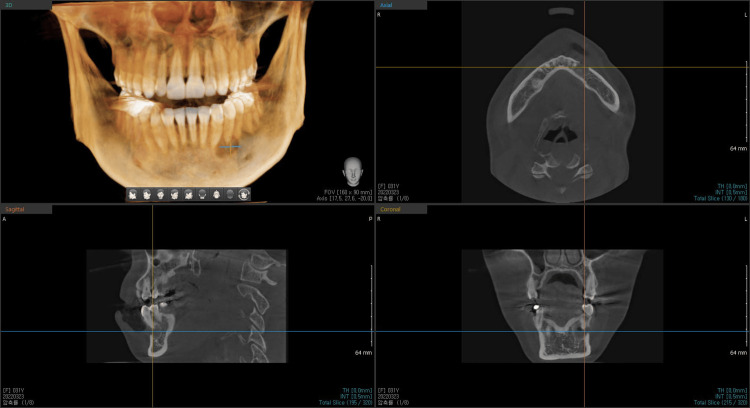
Dental cone beam computed tomography (CBCT) of the lesion 3D reconstruction view and multiplanar view of the lesion
